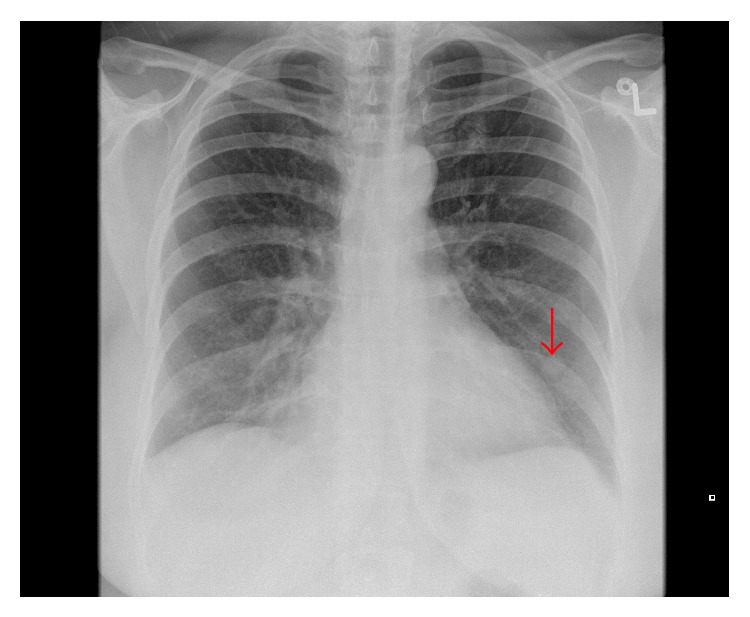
PA chest radiograph: there is a 1.3 cm nodule within the left lower lobe (arrow), projected lateral to the left cardiac border.
